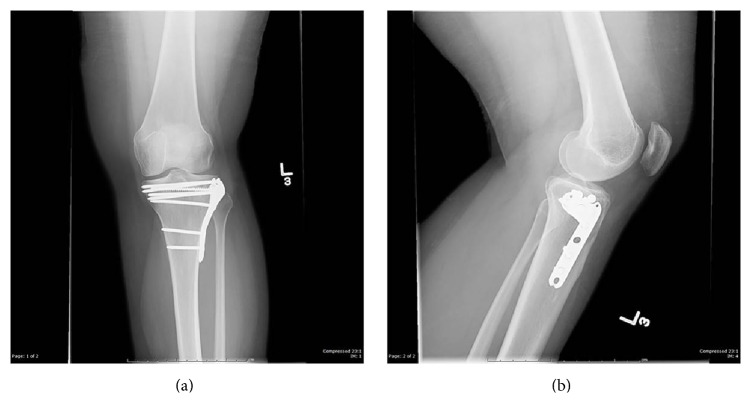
AP and lateral plain radiographs at sixteen months postoperatively.
